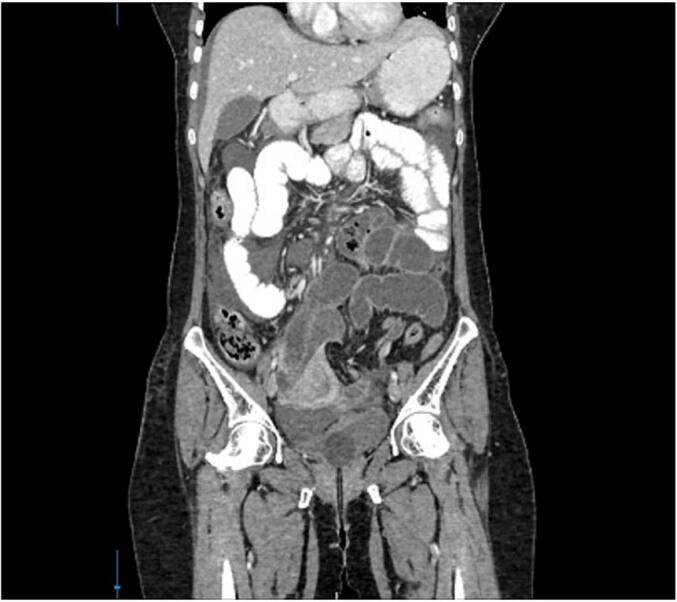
Contrast enhanced CT with small bowel obstruction.
